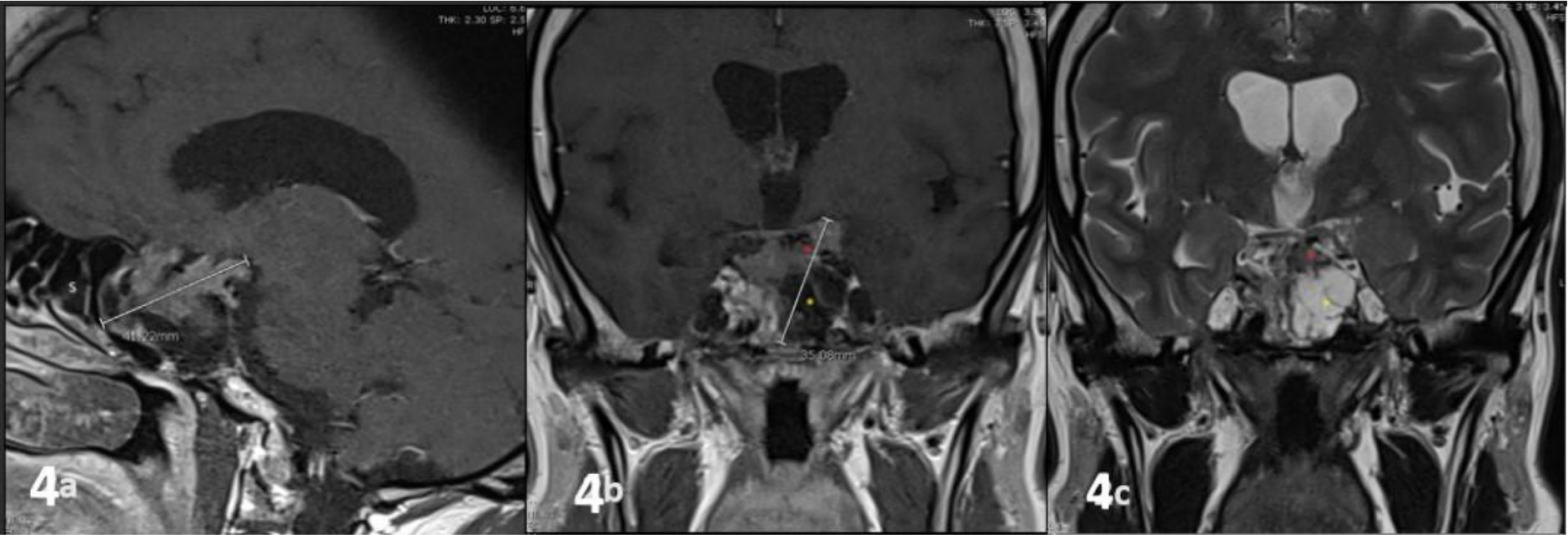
T1-weighted sagittal (a) and coronal (b), and T2-weighted coronal (c). Magnetic resonance imaging of the pituitary gland with intravenous contrast (post-treatment). There is no significant change in the size of the pituitary macroadenoma compared to the pretreatment pituitary MRI.
